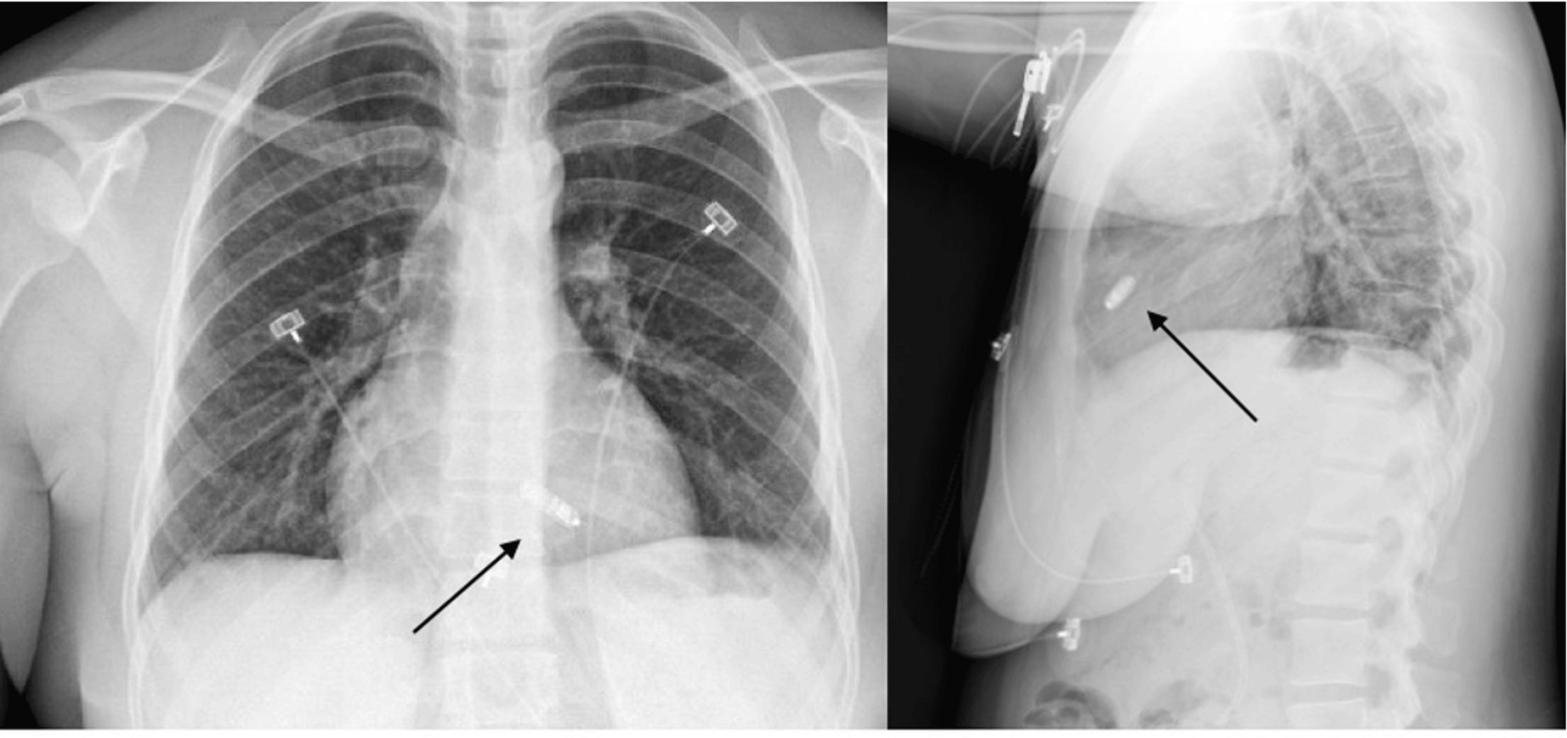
Chest X-ray following pacemaker implantation (arrows) demonstrating appropriate placement.
